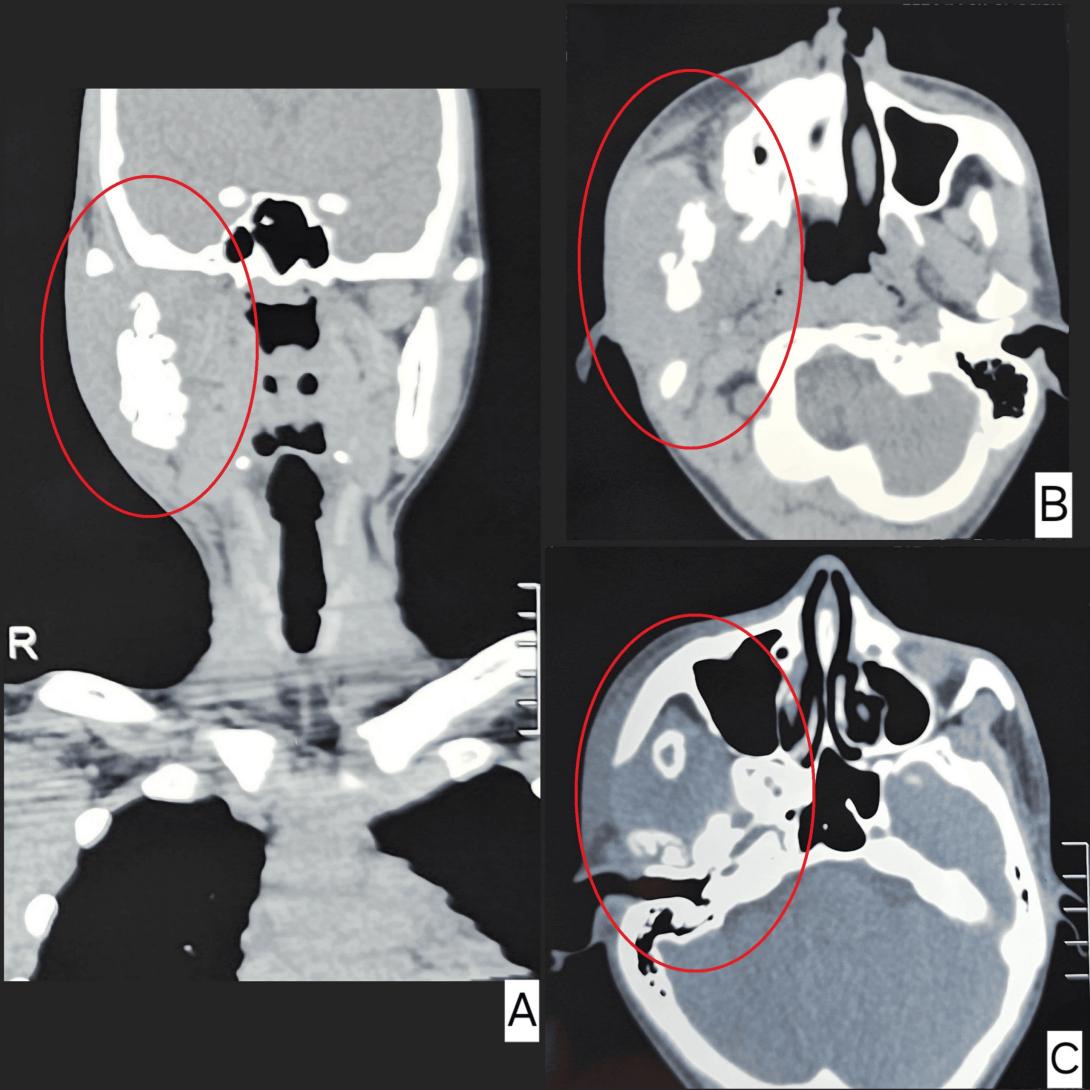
Computed tomography scan taken at the five-day follow-up. Interval decrease in irregularly marginated soft tissue dense lesion with persistent signs of adjacent bone inflammatory changes (A and B). No evidence of any ill-defined hypodense regions within the soft tissue dense lesion to suggest active abscess formation or ongoing necrotic changes (B). Significant resolution of adjacent soft tissue swelling, ipsilateral pre-maxillary swelling, and effacement of the retro maxillary fat pad (B and C). Interval decrease in involvement of infratemporal fossa and involvement of infrazygomatic part of right temporalis muscle - superiorly (C). Persistent signs of involvement in the masseter muscle and skin-laterally, medial and lateral pterygoid - medially, effacement of the ipsilateral para pharyngeal fat pad and likely causing abutment of adjacent torus tubarius with effacement of the ipsilateral eustachian tube (B and A). The above features are suggestive of a significant treatment response with an interval decrease in the soft tissue density lesion.
